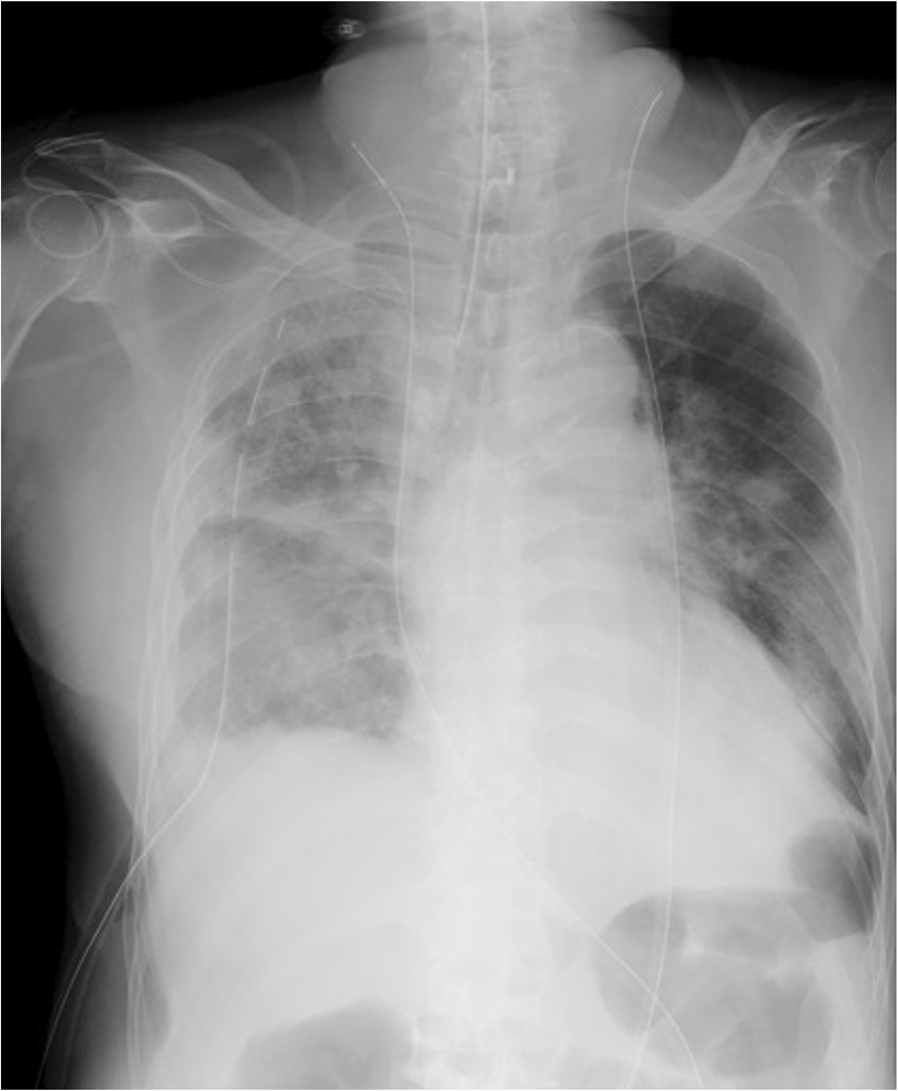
Chest X-ray depicting diffuse bilateral pulmonary infiltrates predominantly in the right lung with pleural effusion after tracheal intubation on intensive care unit day 5
